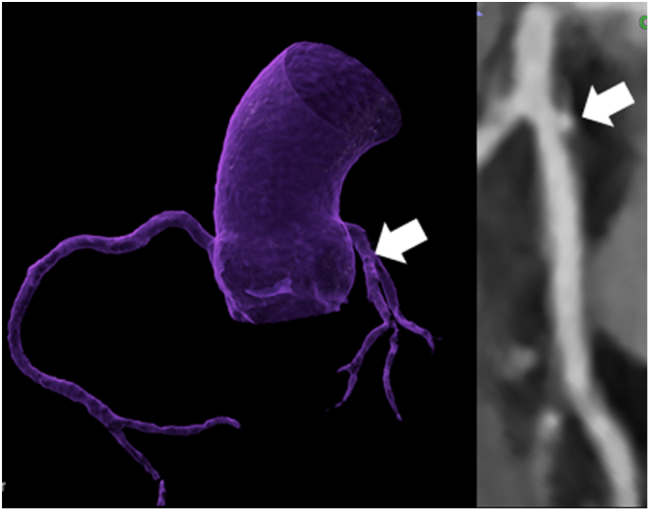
59-year-old male who underwent CTA pre-LT, mixed (predominantly noncalcified) plaque in the proximal and mid LAD with the napkin-ring sign (NRS) with recurrence of hepatitis C–associated liver cirrhosis prior to liver transplantation, MELD Score 21, metabolic syndrome with diabetes, obesity (BMI 32.4 kg/m^2^) and with 9 years survival after LT. CTA = computed tomography angiography; LAD, left anterior descending; LT = liver transplantation.
